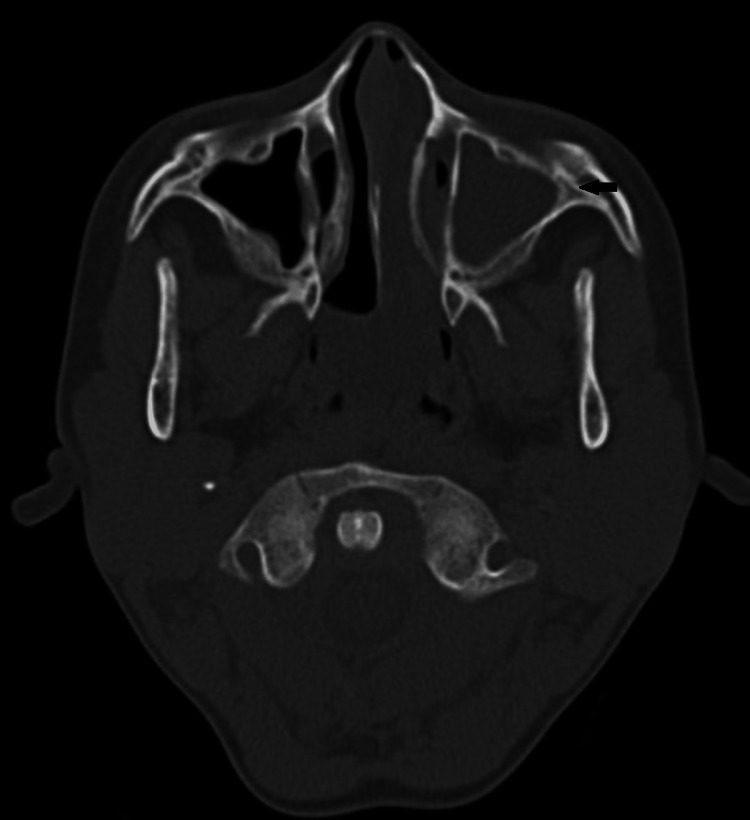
Axial CT scan showing complete haziness of the left maxillary sinus and nostril with thinning of the nasal septum CT: computed tomography
